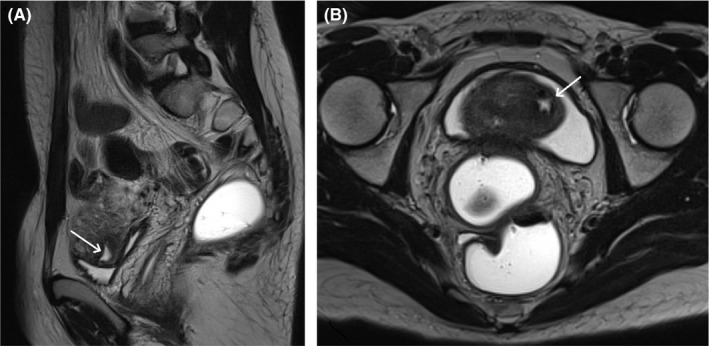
Magnetic resonance imaging: A, a para‐axial view of the left lateral boundary of the uterus (T2 sequence). The white arrow indicates the ACUM (a hyperdense cavity). B, a cross‐sectional view (T2 sequence). The white arrow indicates the ACUM (a hyperdense cavity)
